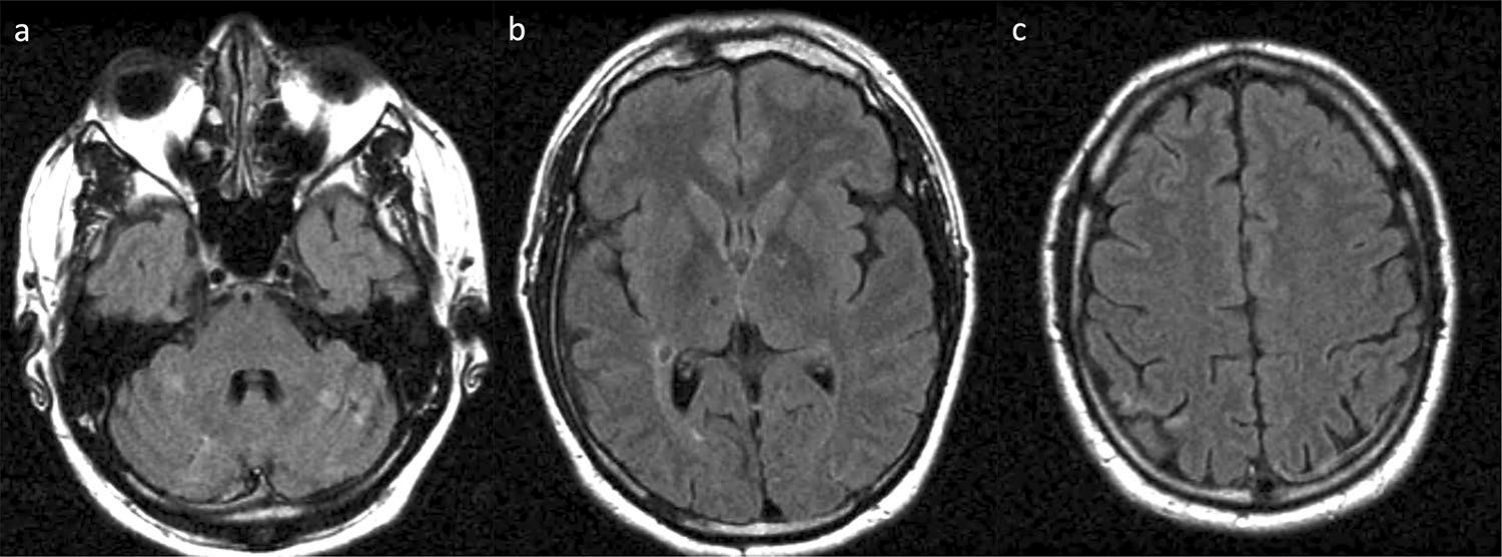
Fabry disease in a 35-year-old, male patient with a history of headache. a–c Axial FLAIR imaging demonstrated multiple, small, old infarcts in the cerebral cortices, deep gray matter, deep white matter, and cerebellar hemisphere
